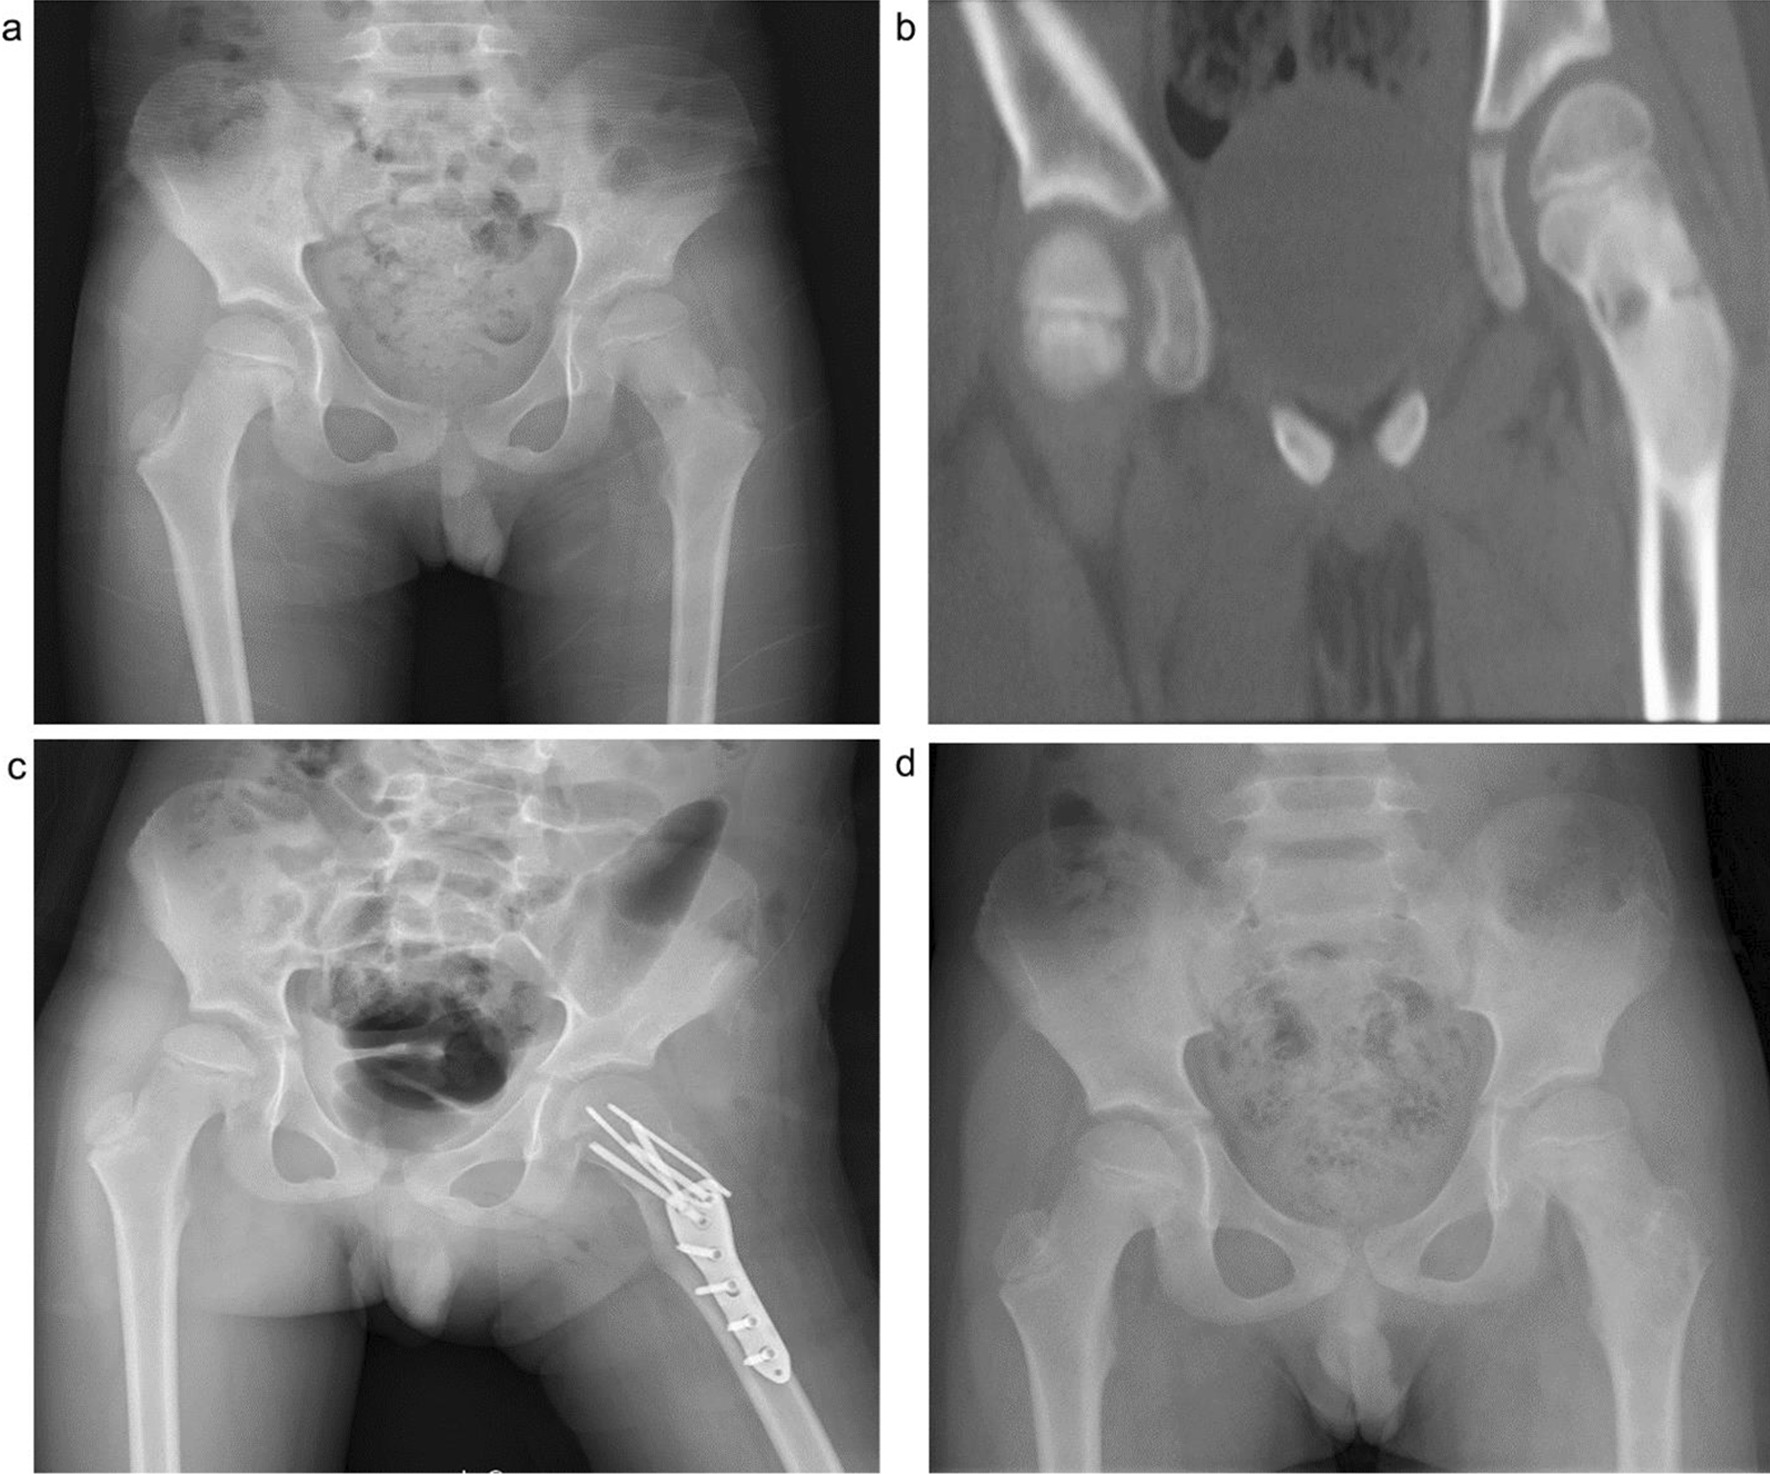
An 8-year-old boy diagnosed with fibrous dysplasia of the left femoral neck with a pathological fracture. Surgical treatment of the left femur tumor was performed by curettage, autogenous iliac bone grafting, and internal fixation with Locking Compression Pediatric Hip Plate™. a. Preoperative X-ray; b. Preoperative CT; c. Postoperative; and d. Two years after operation
